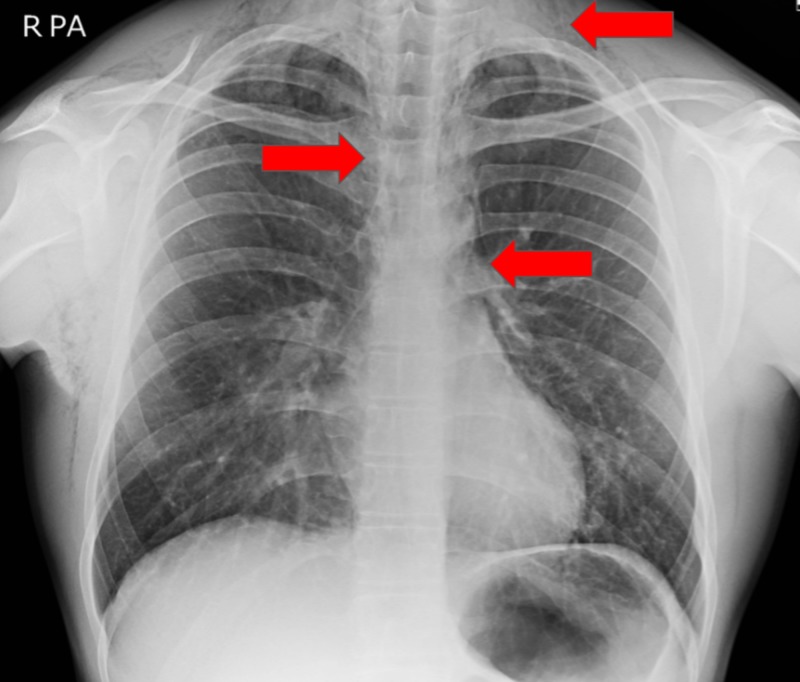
Plain film chest x-ray Pneumomediastinum with widespread air in the mediastinum and extending into the soft tissues of the thorax and neck was noted (red arrows).
